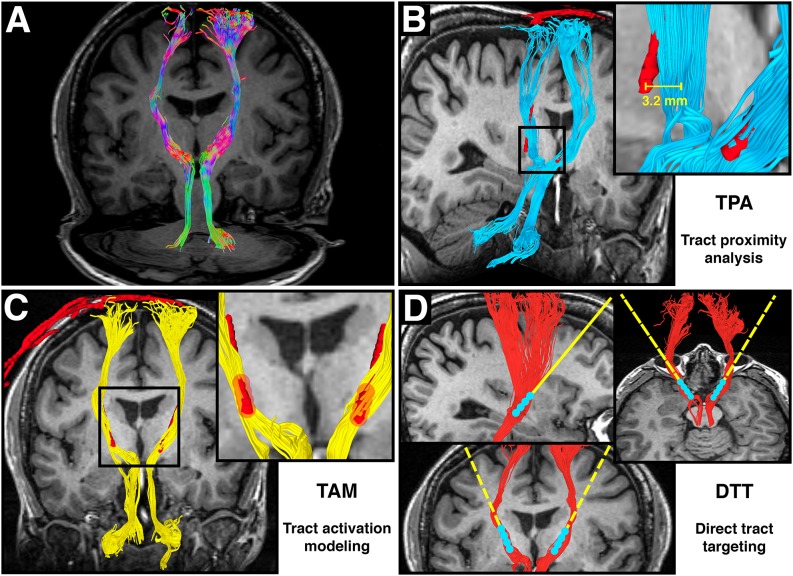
Adapted from Calabrese et al. (2015b) Figure 6, with permission. (A) Directionally colored diffusion MRI tractography (DT) of the dentatorubrothalamic tract (DRT), which connects the dentate nucleus of the cerebellum, red nucleus, ventral intermediate thalamus, and motor cortex. Note the absence of a midline crossing in the midbrain, which is a limitation of the diffusion tensor imaging (DTI) technique. (B) Tract proximity analysis (TPA). Distances between the DRT (blue) and the deep brain stimulation (DBS) electrode (red) are compared with treatment outcomes. (C) Tract activation modeling. Fiber tracts (yellow) are generated from a region of interest surrounding the DBS contacts (red). (D) Direct tract targeting (DTT). The DT model of the DRT (red) is used for preoperative DBS electrode targeting.
